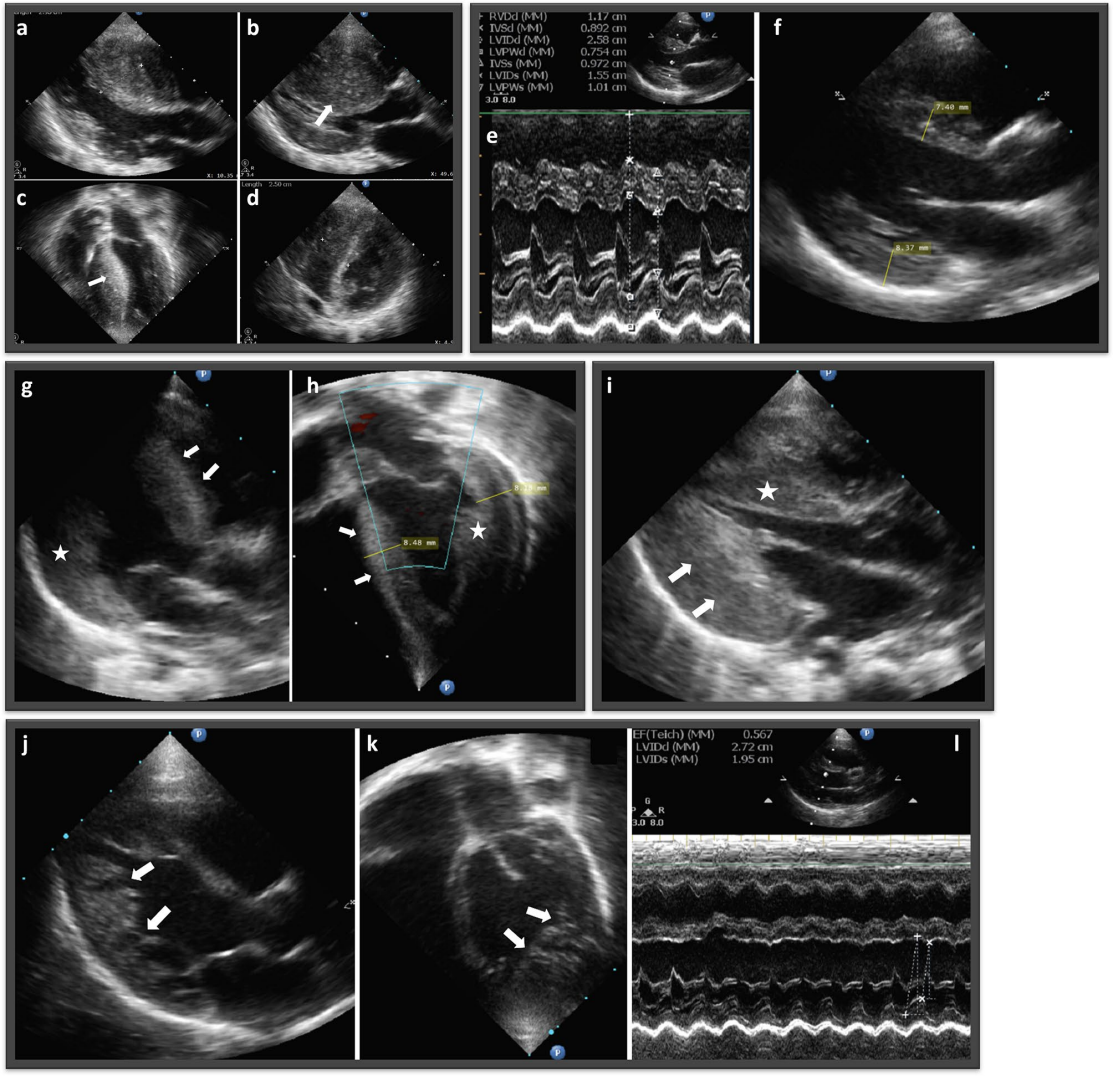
Echocardiographic images of patients with HCM and LVNC. (a–d) Patient in family F01. (a,b) Parasternal image in diastole and systole, respectively, showing the severely hypertrophic interventricular septum with diastolic dimension of 2.93 cm, and almost obliteration of the left ventricular lumen in systole. (c) is an image of the four-chamber view, and (d) of the short access view of the left ventricle showing the eccentric hypertrophy involving predominantly the interventricular septum. (e,f) Family 02 proband with hypertrophic cardiomyopathy. (e) M-mode echocardiography showed concentric hypertrophy with diastolic dimensions of the interventricular septum and posterior wall of 7.5–8.9 mm and preserved systolic function. (f) Two-dimensional echocardiographic image of the left ventricle showing hypertrophy of both septum (7.4 mm), and posterior wall (8.4 mm), with smooth, well-compacted myocardium. (g,h) Echocardiographic images of patient in family F03. (g) is a parasternal view, and (h) is an apical view of the left ventricle showing concentric left ventricular hypertrophy involving both the septum (white arrows) and the posterior wall (white star), the endocardial aspect is smooth, and with no evidence of left ventricular non-compaction. (i) Proband of Family F04. longitudinal echocardiographic image of the left ventricle in diastolic phase, showing severe hypertrophy of both interventricular septum (white star), and posterior wall of the left ventricle (white arrows), with well-compacted myocardium. (j–l) Proband in family F08. (j,k) Parasternal and apical views of the left ventricle showing non-compaction involving the apex and the posterior wall of the left ventricle (white arrows), and sparing the interventricular septum. (l) M-mode echocardiography showing mildly depressed left ventricular systolic function.
